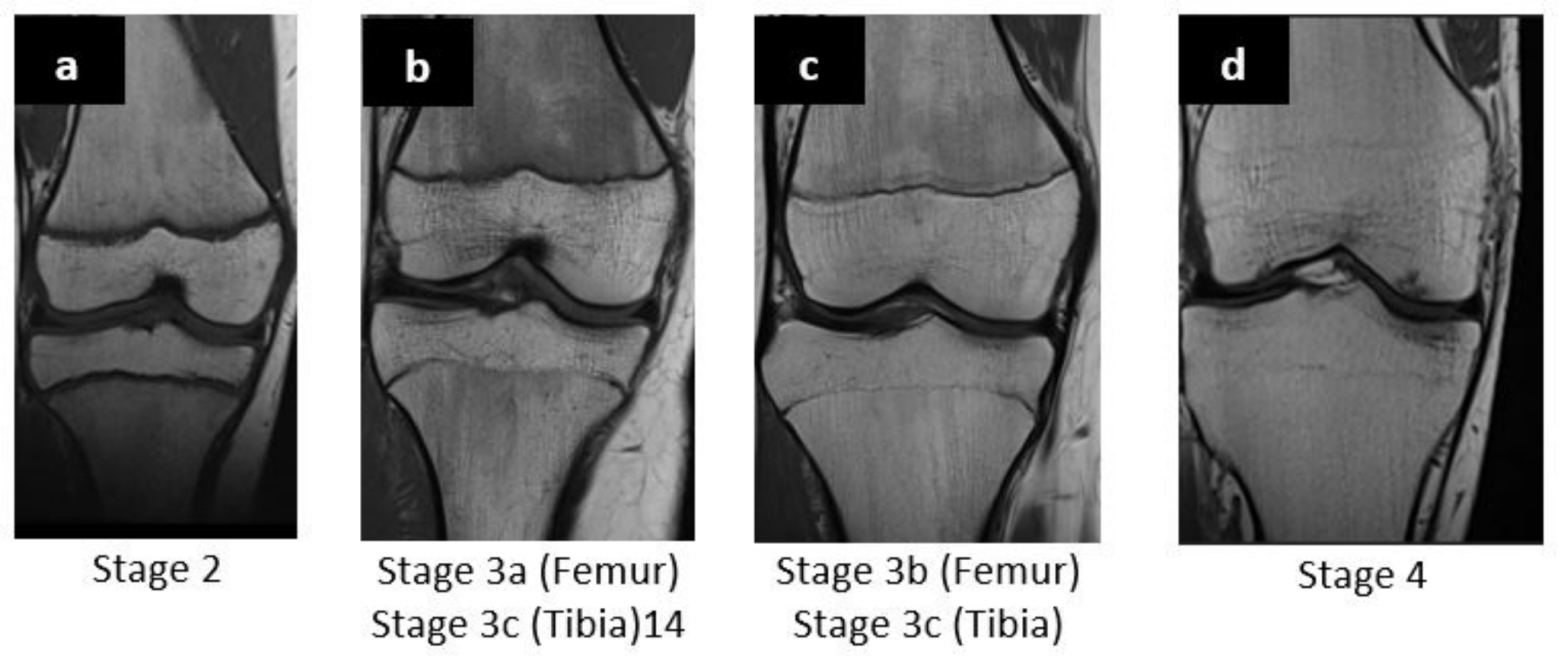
Coronary MRI images in T1-TSE sequence. a: Distal femur and proximal tibia of a 10-year-old female patient in stage 2. b: Distal femur and proximal tibia of a 14-year-old-female patient. c: Distal femur and proximal tibia of a 17-year-old male patient in stage 3b. d: Distal femur and proximal tibia of a 21-year-old male patient in stage 4. MRI: Magnetic Resonance Imaging, TSE: Turbo Spin Echo
